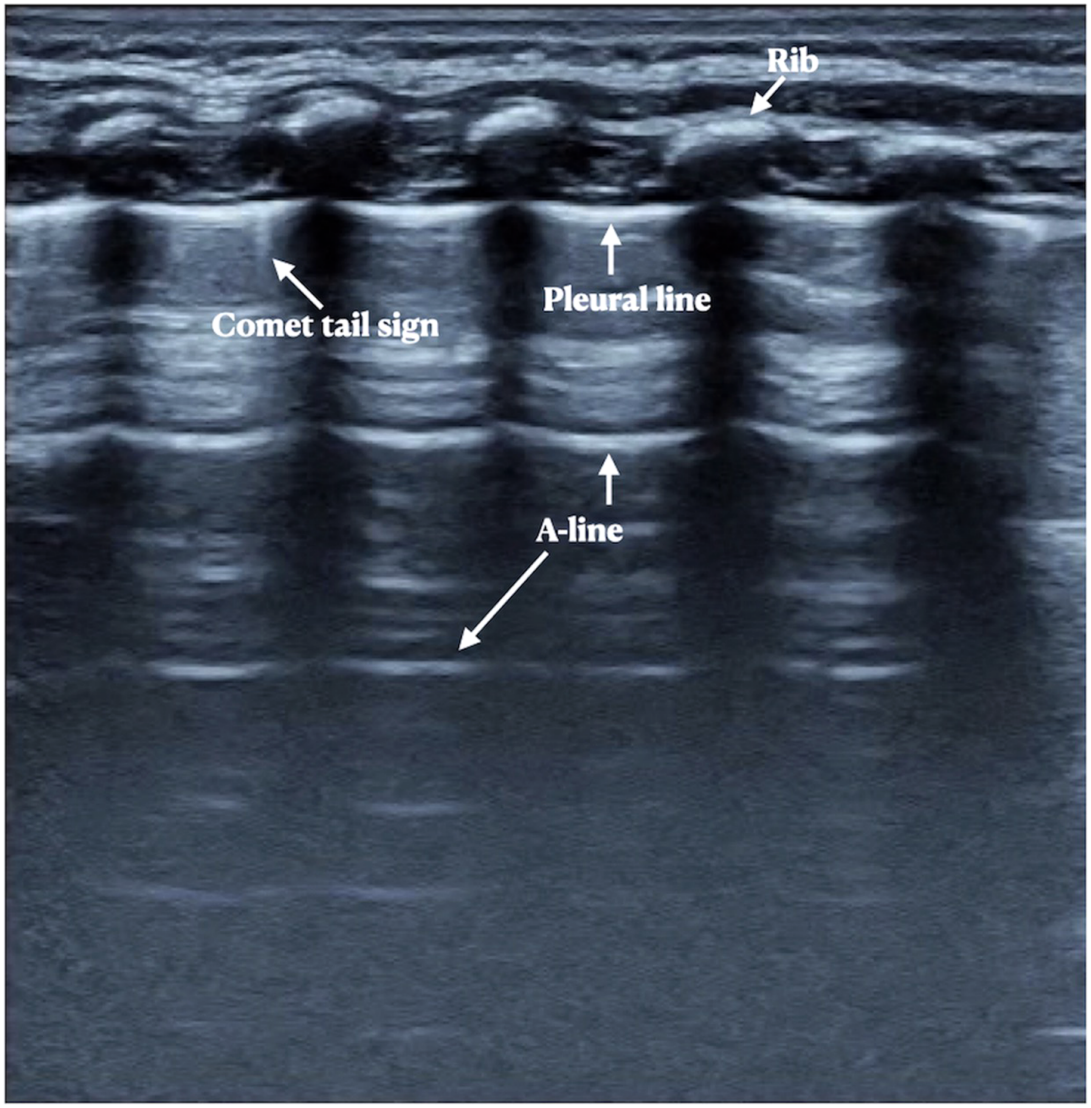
Neonatal normal lung ultrasound findings. On B-mode imaging, the neonatal normal lung presents as the bamboo sign. The pleural line and A-line present as smooth, regular and hyperechoic lines arranged in parallel and equidistant from each other, and the A-line echoes gradually diminish until disappeared. In those infdants less than a week after birth, a few B-lines or comet tail signs may sometimes be seen, but there is no pleural effusion or lung consolidation.
